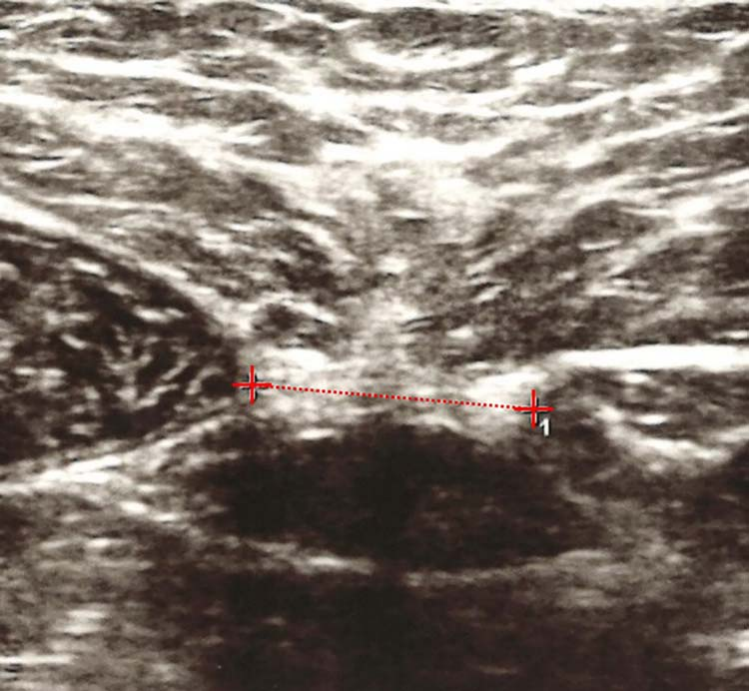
Interrecti distance (IRD) measurement using ultrasound imaging. The line from which the IRD was measured is indicated by a dotted line.
